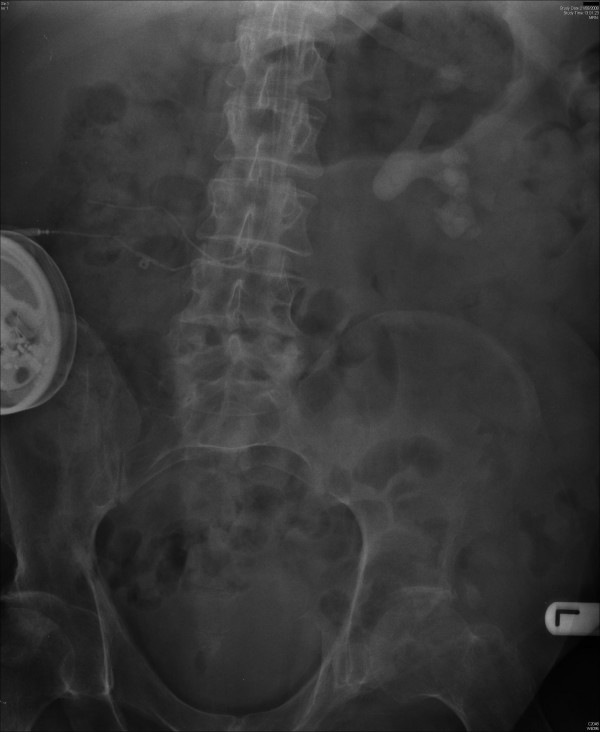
X-ray of kidneys, which was taken on 21 August 2008, showed stag horn calculus with branches in upper, middle and lower calyces.
